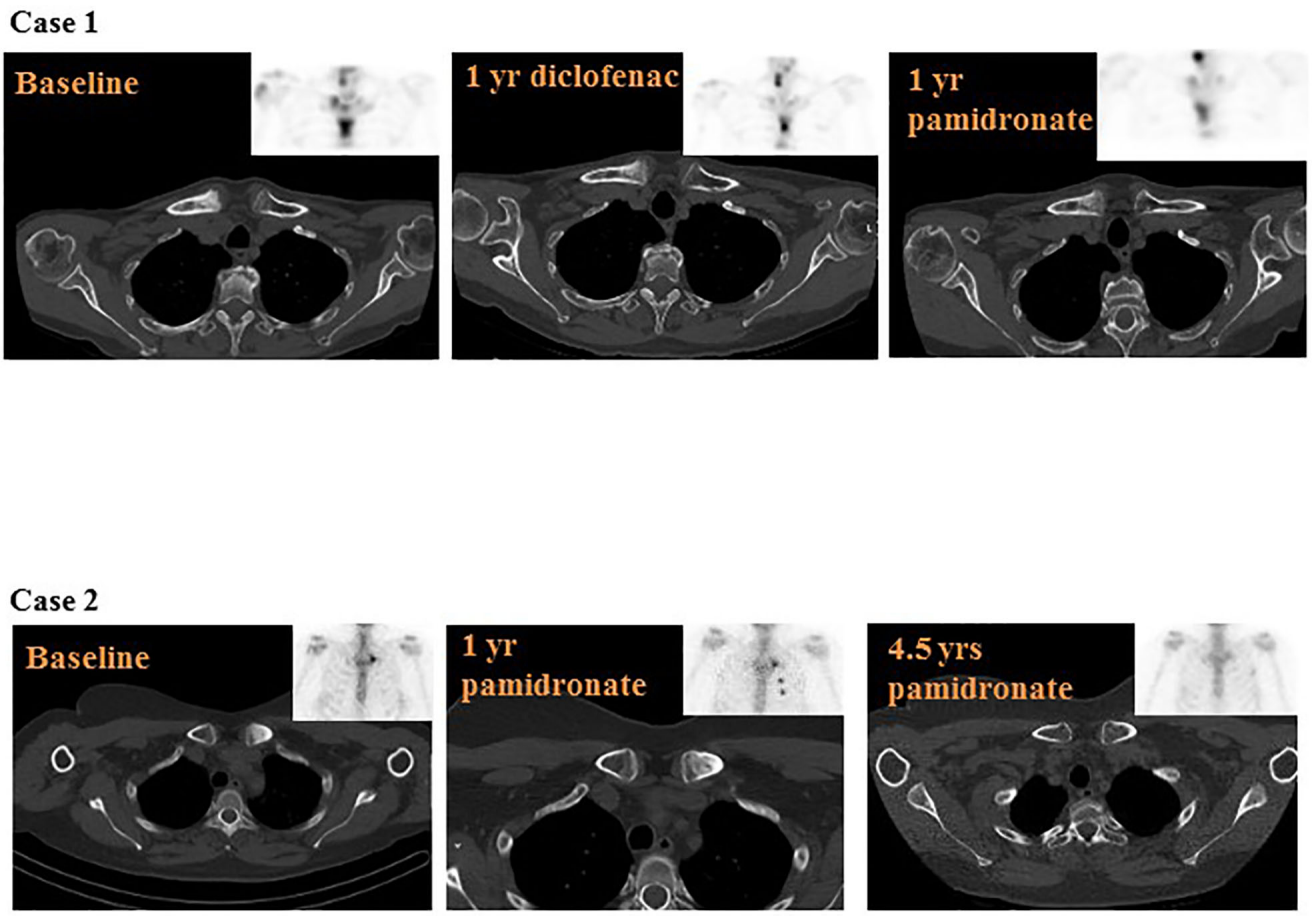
Overview of disease course on computed tomography (CT)-scan and skeletal scintigraphy. Legend: Case 1: Baseline: diffuse sclerosis of the right clavicular end with increased bone turnover, and increased bone turnover of the manubrium and proximal corpus sterni. 1 year diclofenac: persistent sclerosis and increased bone turnover of the right clavicular end and persistent increase of bone turnover of the manubrium and proximal corpus sterni, slightly reduced compared to baseline. 1 year pamidronate: unchanged sclerosis and reduction of bone turnover of the right clavicular end, the manubrium and proximal corpus sterni, compared to baseline. Case 2: Baseline: sclerotic changes of the left clavicular end with elevated bone turnover. 1 year pamidronate: slightly reduced sclerosis of the left clavicular end and slightly reduced bone turnover. The increased uptake of the 3^rd^ and 4^th^ rib was caused by two costal fractures. 4.5 years pamidronate: almost complete resolution of sclerosis, leaving a minimal rest, and complete normalization of bone turnover.
